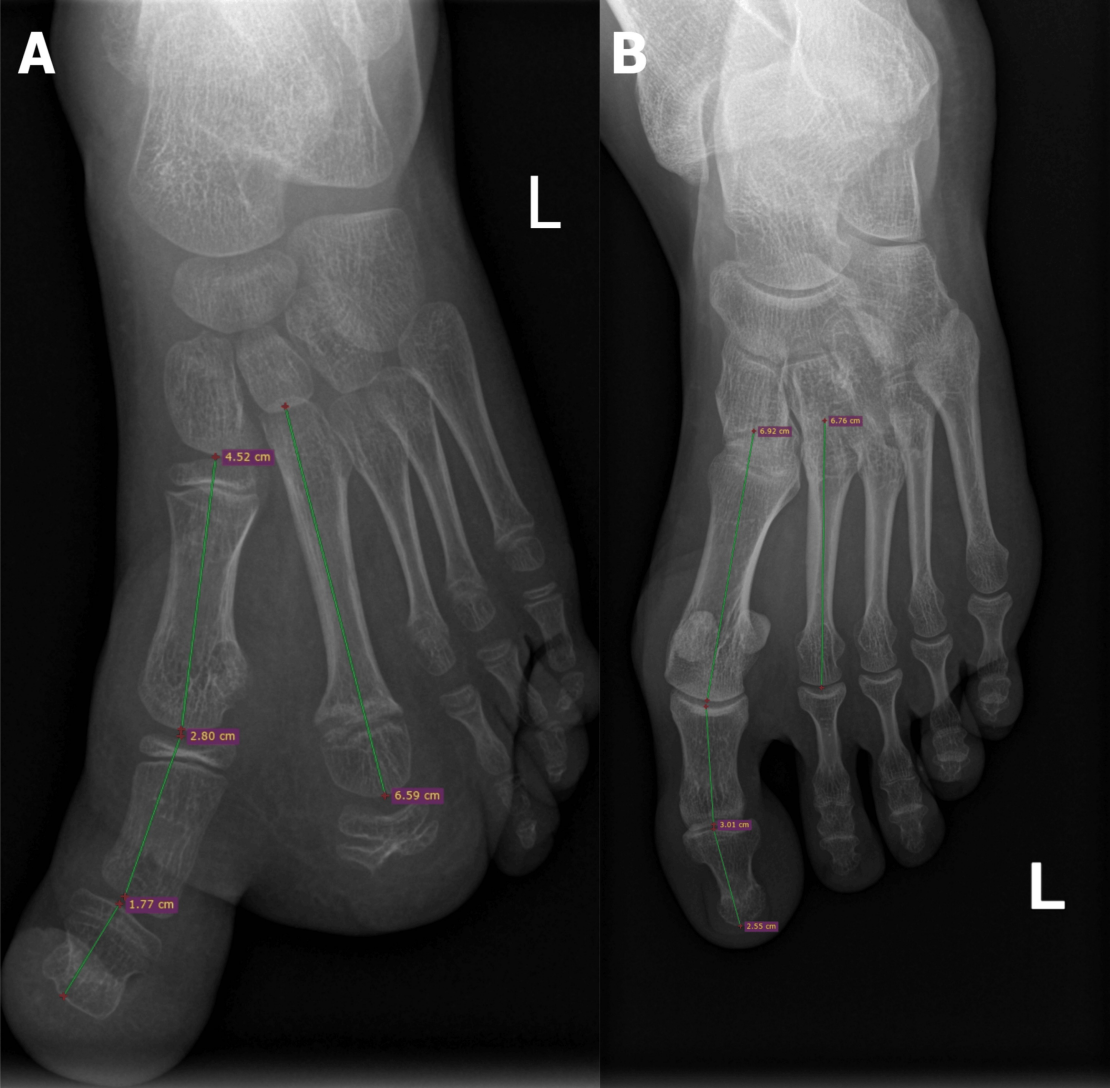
Antero-posterior (AP) X-ray of the patient's left foot at six years of age (A) compared to the left foot of the girl's mother (B). Patient's left foot first metatarsal, hallux, and second metatarsal measurements (A) compared to the mother's left foot (B).
